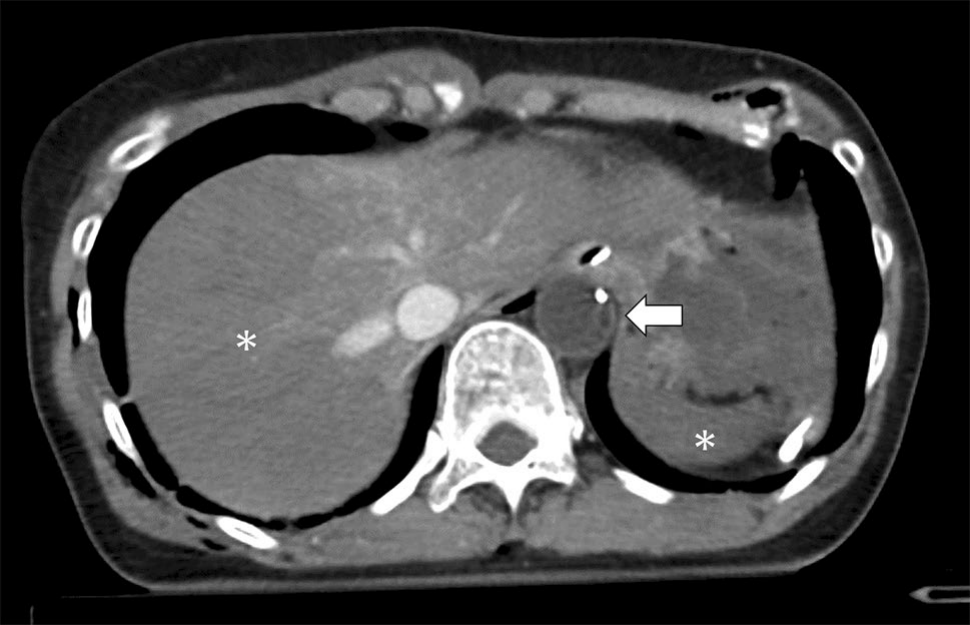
Abdominal contrast-enhancement computed tomography (venous phase) with complete Zone I resuscitative endovascular balloon occlusion of the aorta (arrow) showing hypo-enhancement (hypoperfusion) in the liver and spleen (asterisks)
